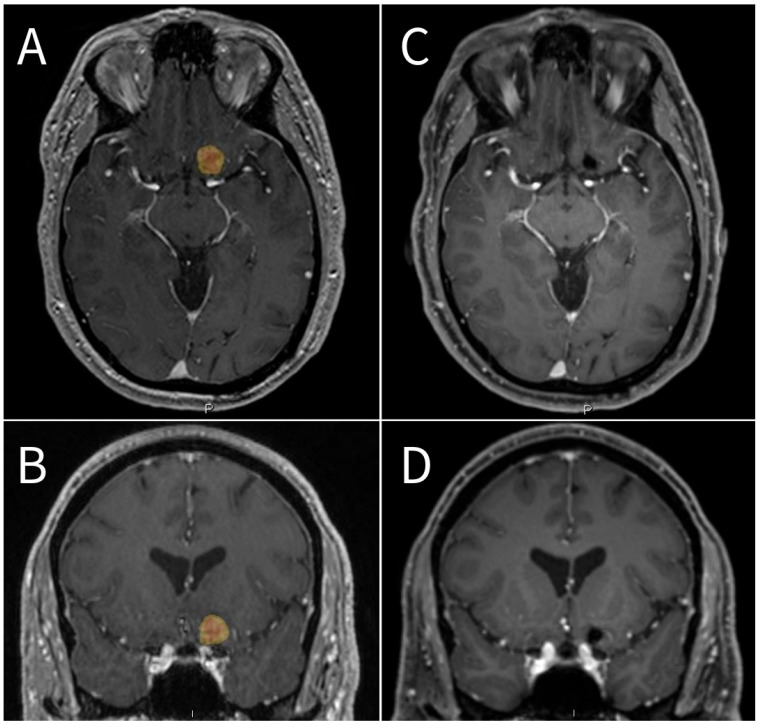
A 54-year-old male patient with BRAF V600 mutation positive melanoma on maintenance with nivolumab was diagnosed with a new left inferior frontal lobe metastasis adjacent to the left optic nerve and measuring approximately 17 mm in size. The patient was offered hypo-fractionated Gamma Knife radiosurgery in an attempt to reduce the risk of radiation-induced optic neuropathy. (A) Axial and (B) coronal, T1-weighted, post-contrast, procedural brain MRI showing the 17 mm left inferior frontal lobe lesion in close proximity to the optic apparatus. The patient was treated with a prescription dose of 25 Gy at the 50% isodose line (yellow overlay), delivered over 5 fractions. The maximum point dose per fraction delivered to the left optic nerve was 3.5 Gy. (C) Axial and (D) coronal T1-weighted, post-contrast, 30-month follow-up brain MRI showing complete tumor response. “P” for posterior and “I” for Inferior.
